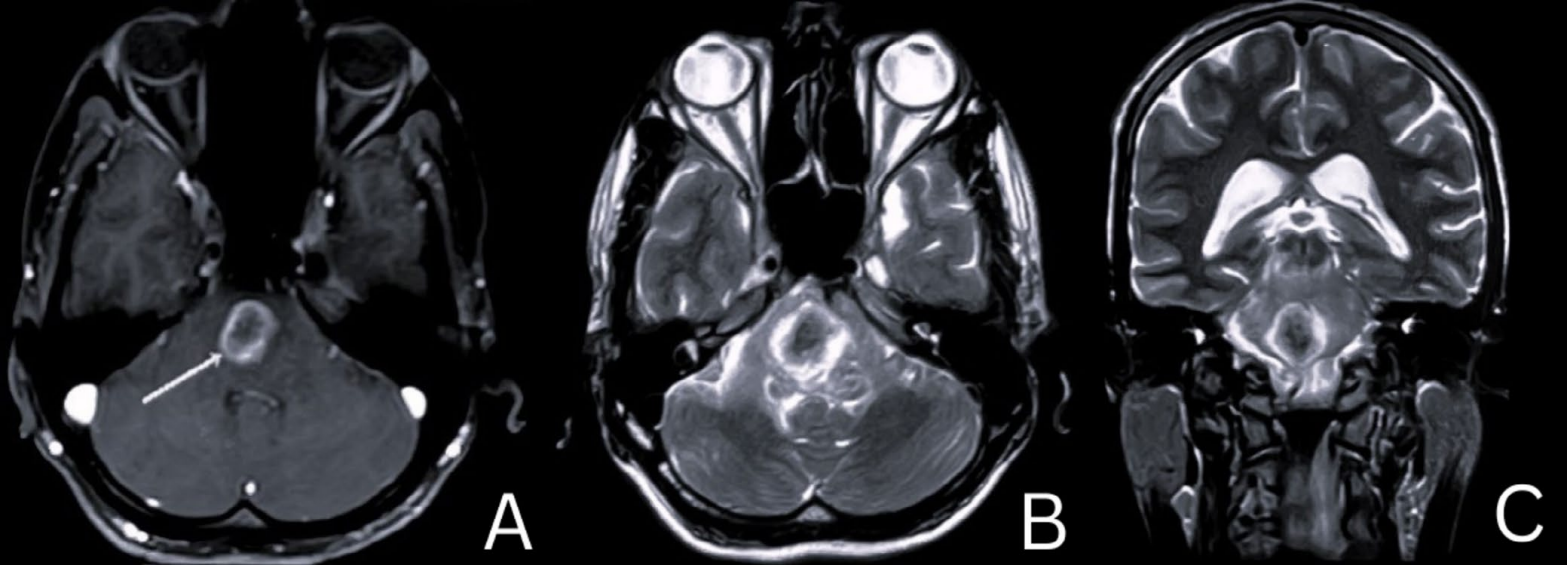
Gadolinium contrast‐enhanced magnetic resonance imaging (MRI) of the brain, (A) T1‐weighted axial image and T2‐weighted (B) axial, and (C) coronal view images showing a well‐defined ring‐enhancing lesion measuring 1.8 × 2.0 × 2.1 cm at the level of the pontomedullary junction with surrounding perilesional vasogenic edema.
